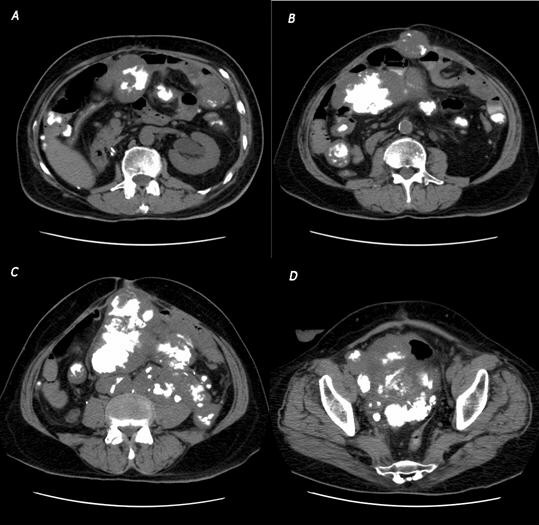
CT appearance at multiple cuts (A – D) of the recurrent tumor. The recurrence was more homogeneous than the primary tumor, consisting almost completely of the calcific component of the nonlipomatous portion of the tumor.
